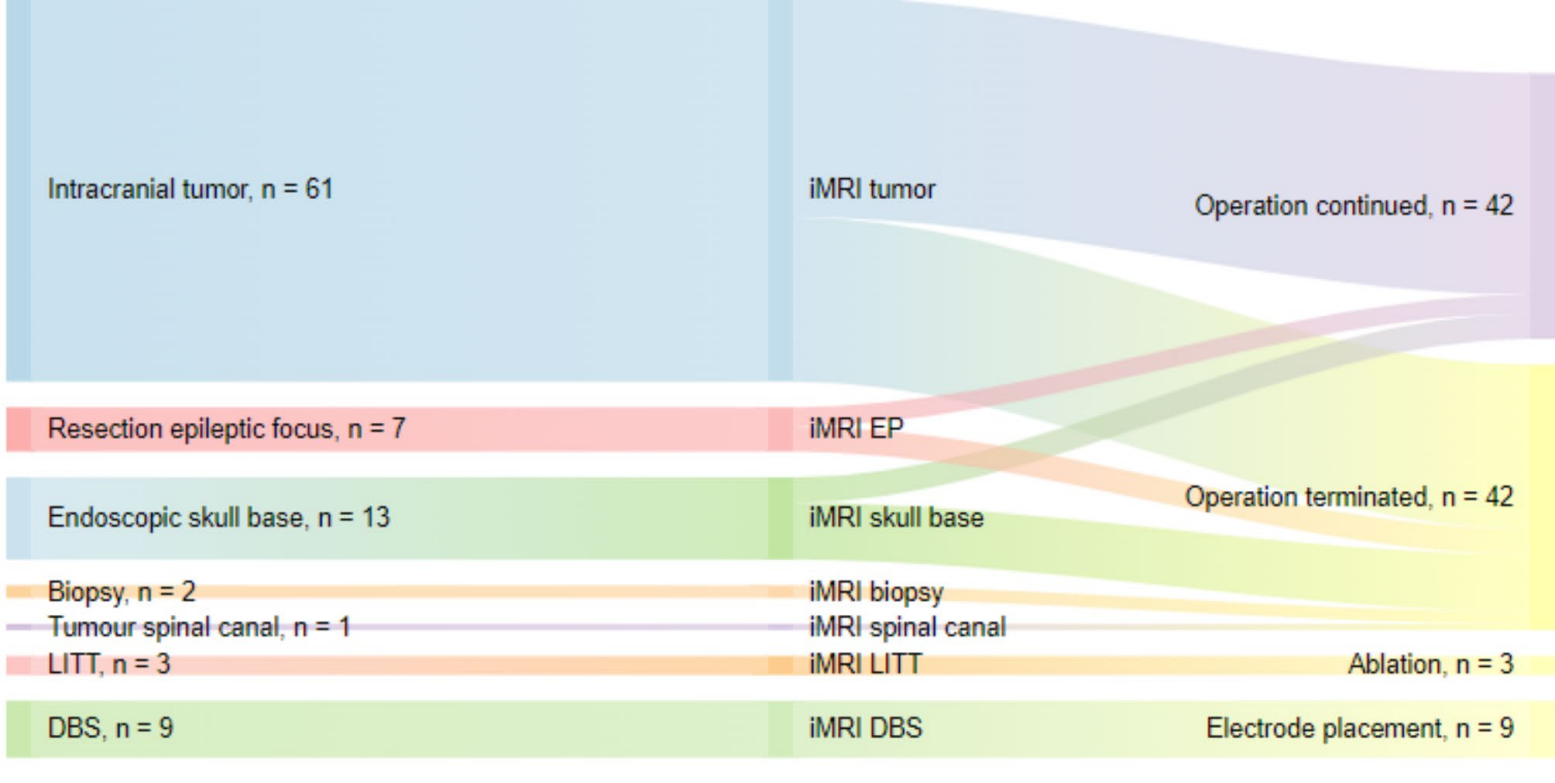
Sankey plot over performed surgeries and whether surgery was continued or not (*n* = 96). Patients undergoing endoscopic skull base surgeries with pituitary/skull base lesions who did not perform an intraoperative MRI (4) are excluded in Fig. 3
